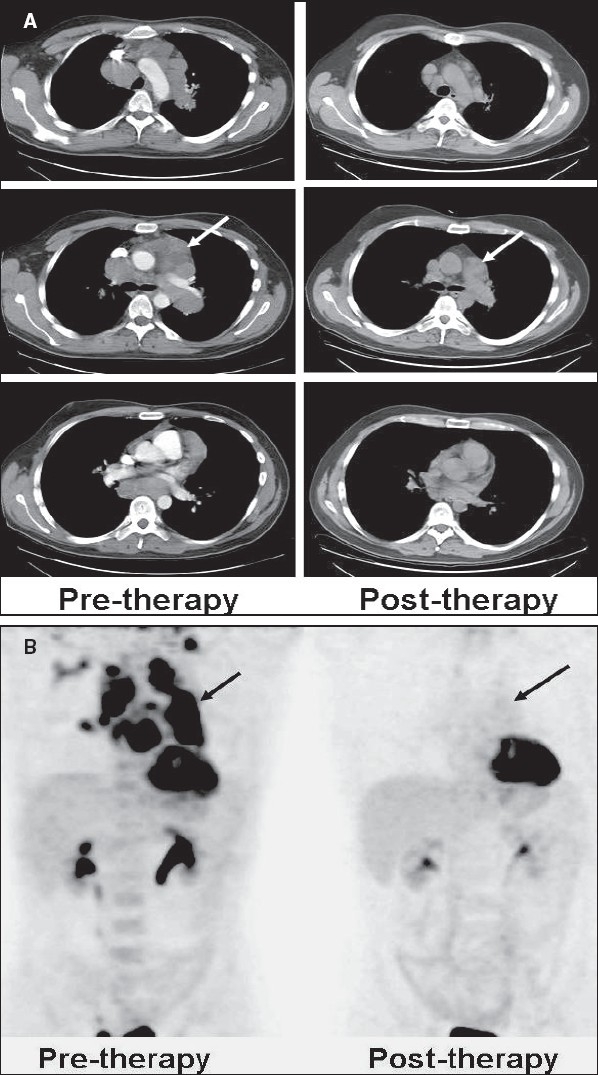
Pre-therapy (left) and post-therapy (right) axial CT scan images (A) of a patient with lymphoma indicate incomplete response to therapy with residual lymphadenopathy in the mediastinum (arrows). The pre-therapy (left) and post-therapy (right) MIP images (B) from a PET study of the same patient (performed within a week of the respective CT scans) show complete remission (arrows)
